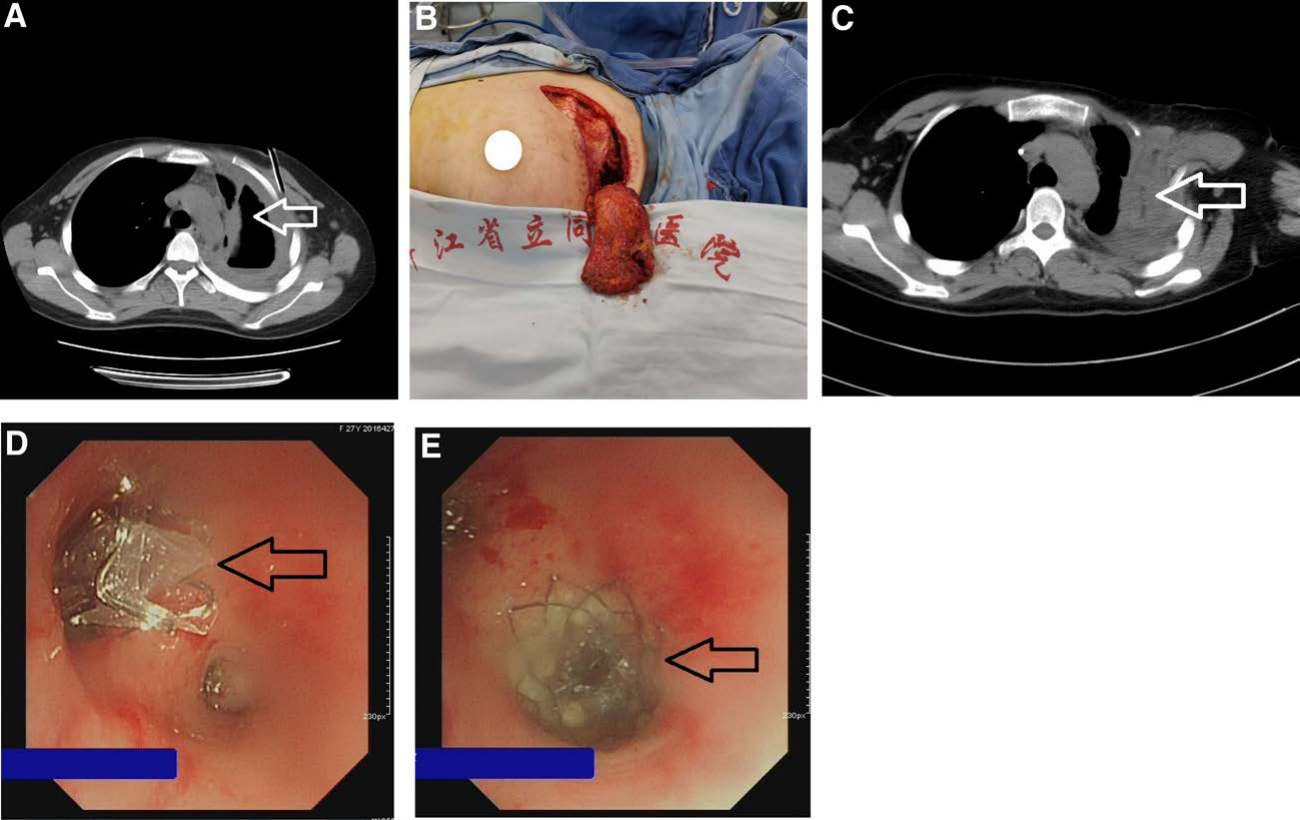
The abscess cavity in the upper field of the left lung of patient A was approximately 90 mL in size (arrow of Figure a, drainage tube can be seen in the anterior chest wall). The abscess cavity was filled with the ipsilateral pectoralis major muscle (Figure b). Chest CT was reexamined 9 days after the operation, and no abscess cavity was found (Figure c). Fistulas of apicoposterius segmentum and anterius segmentum were blocked for a larger bronchial one-way valve (Figure d). Fistula of superior lingular segment was blocked for a smaller one (Figure e).
